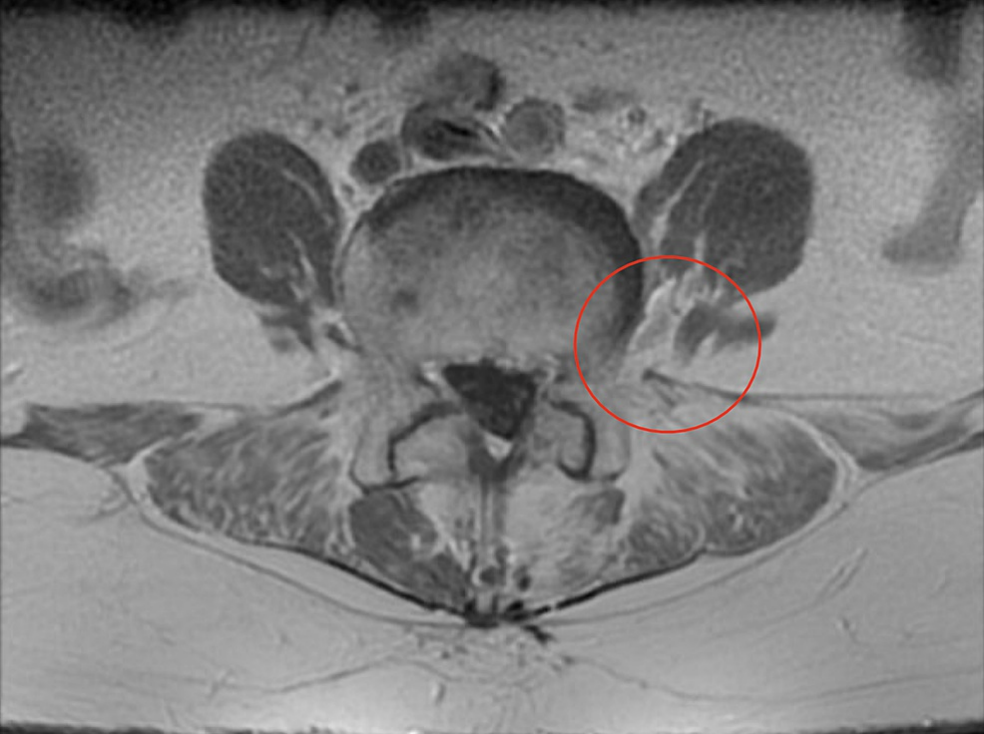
Axial magnetic resonance imaging of the lumbar spine Axial spine MRI demonstrated enhancement of the left L4 nerve roots (as indicated by the red circle) suggestive of inflammation or neuropathy. There was no evidence of masses or impingement on imaging.
